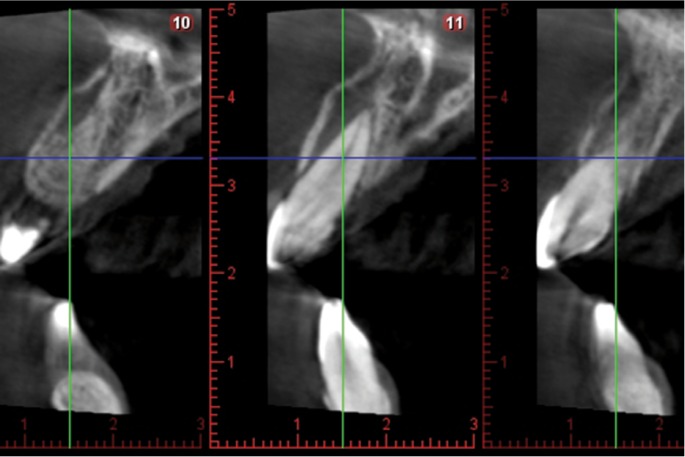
CBCT view showing of the upper central incisors, showing the alveolar fracture of the upper anterior buccal plate.
